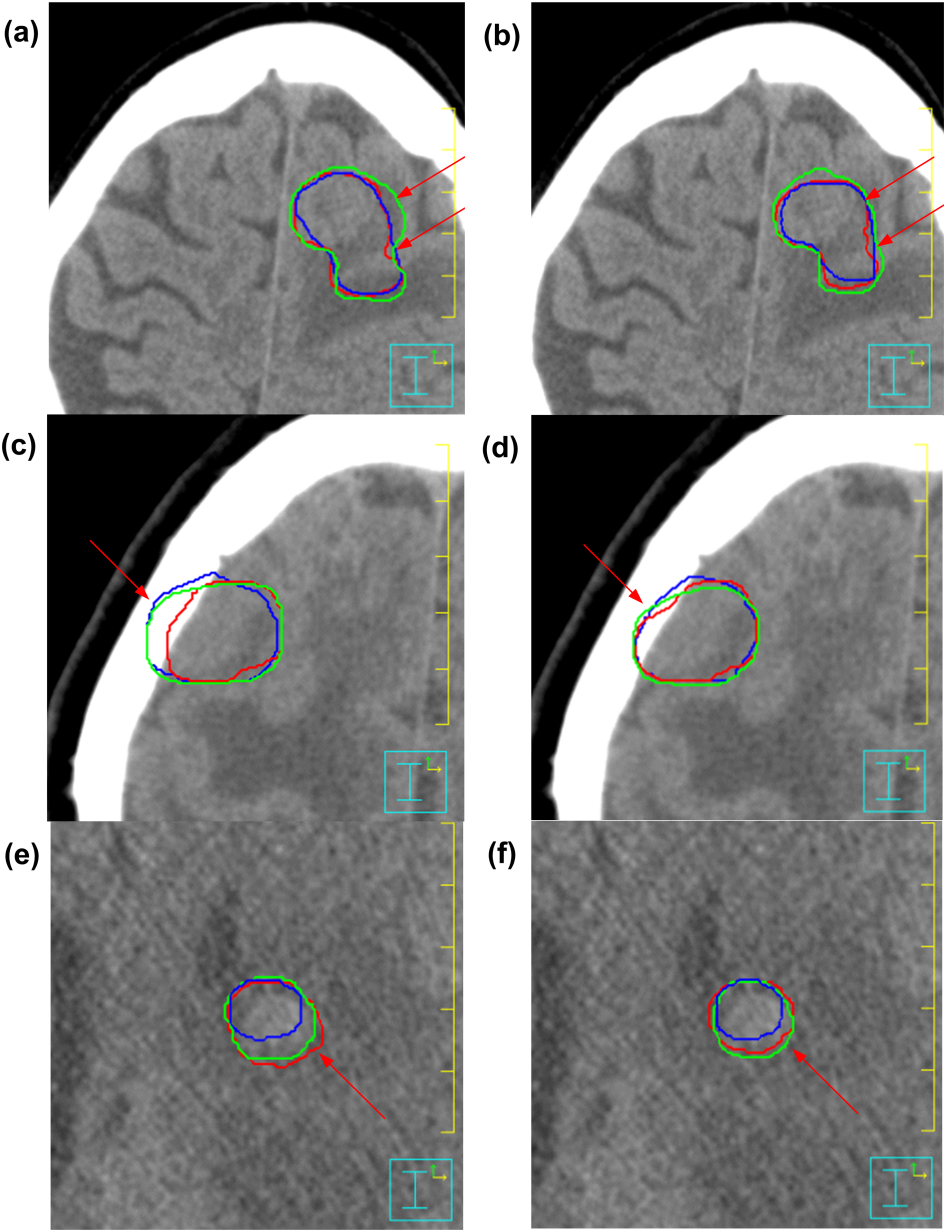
The GTVs delineated on axial slices of patient CT images by the three observers with dual-modality fusion (a, c, e) and tri-modality image fusion (b, d, f) methods, respectively. (a) and (b) are for patient 1; (c) and (d) are for patient 2; (e) and (f) are for patient 3.
